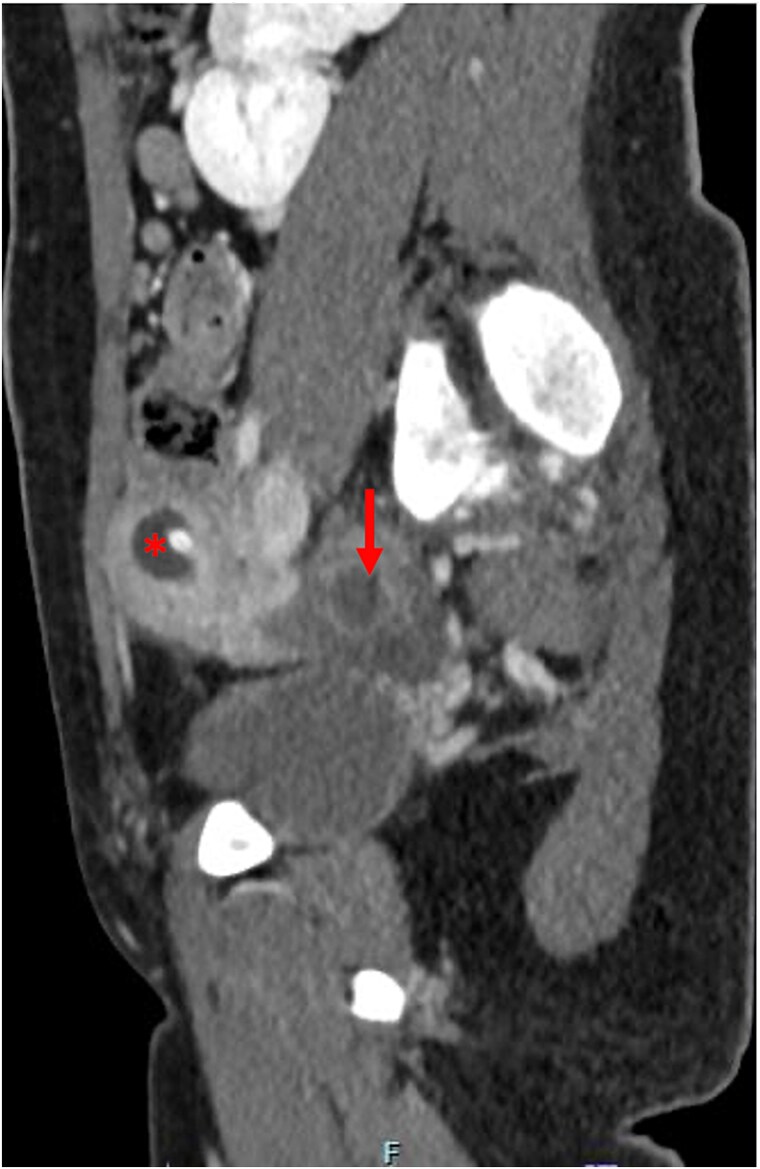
Post-operative computed tomography abdomen/pelvis demonstrating resolution of the hematometra (^*^) and improvement of the hematosalpinx (arrow).
